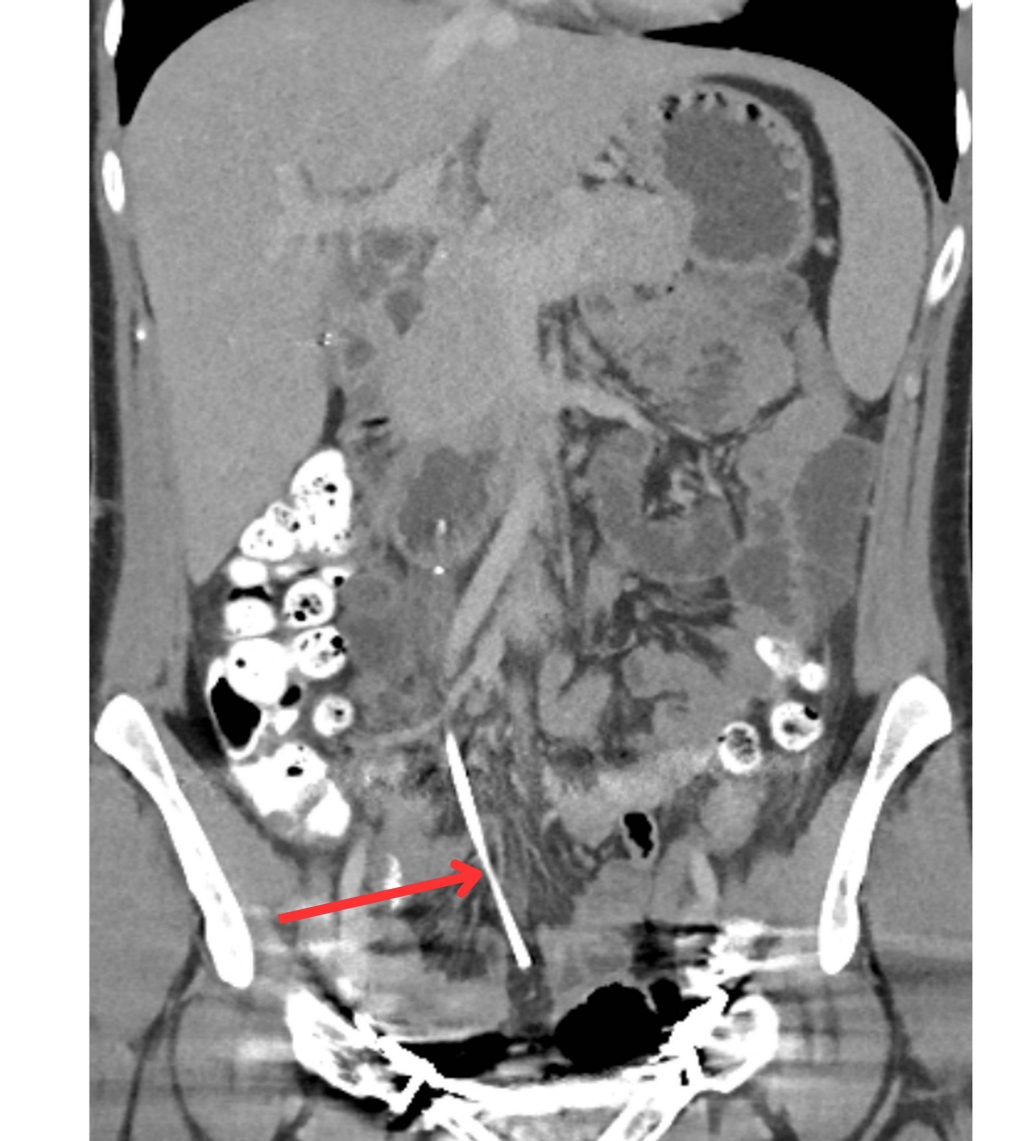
CT of the abdomen and pelvis showing the tip lying in the inferior aspect of the small bowel mesentery near the expected level of the urinary bladder.
